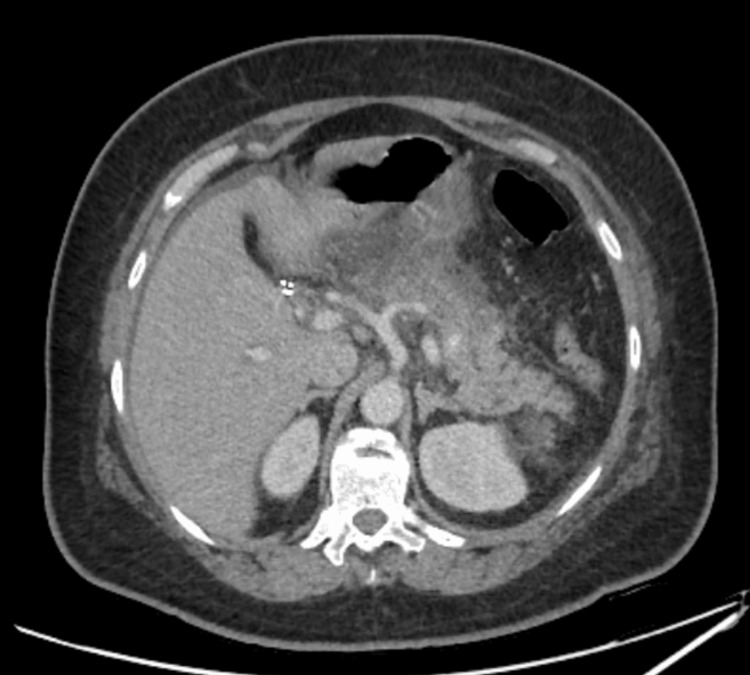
CT scan showing acute pancreatitis.
